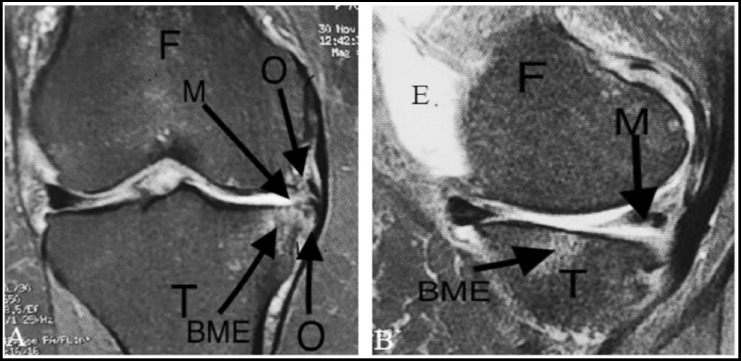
Representative figure for the knee osteoarthritis (KOA) lesions documented by Magnetic resonance imaging (MRI) of the studied population [a- coronal, b- sagittal]. BME, Bone marrow edema; E, Effusion; F, femur; M, Meniscus (post horn medial meniscus) tear; O, osteophytes; T, Tibia..
